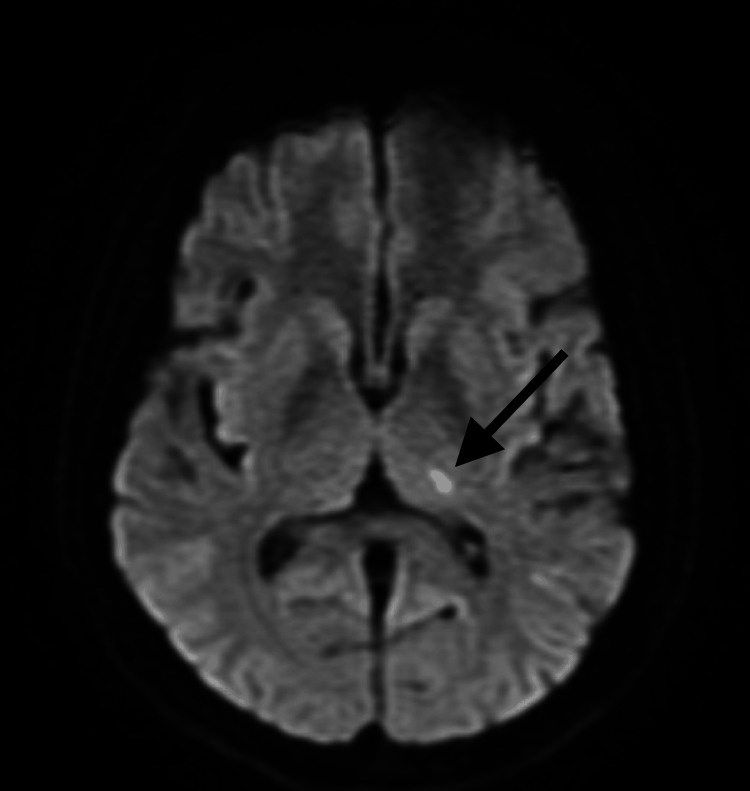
Axial diffusion-weighted imaging (DWI) MRI demonstrating an acute lacunar infarction in the left thalamus (indicated by the black arrow).
